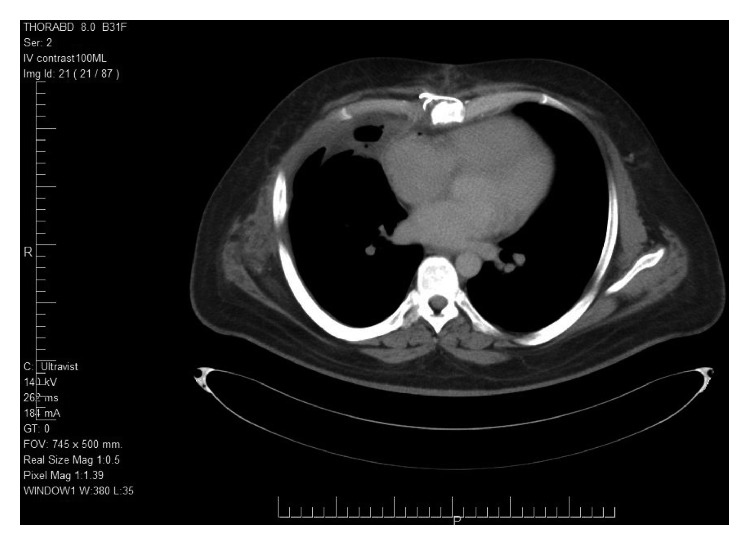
CT scan follow-up postresection of tumor.
